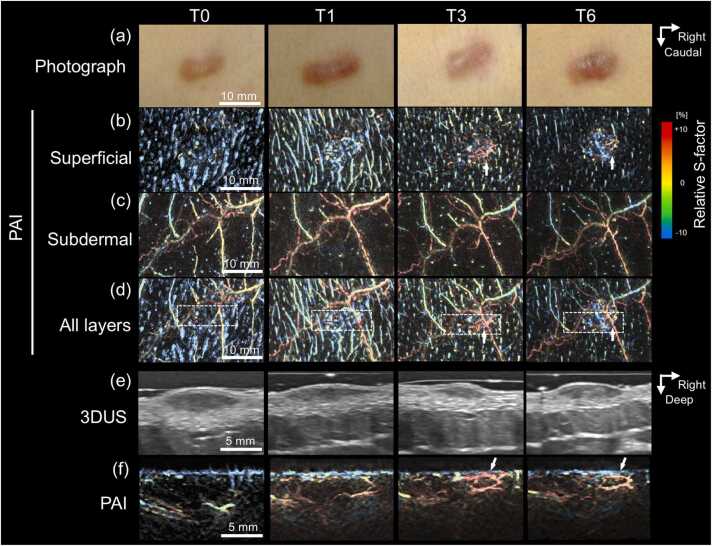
Longitudinal changes in Case 3. The keloid initially exhibited a small, oval morphology (a) with a lenticular profile on ultrasonography (e). The lesion flattened between T0 and T1; however, thickening was observed between T3 and T6. Photoacoustic images with color-coded relative S-factor values reveal no clusters of microvessels in the superficial layer (b), whereas several veins with heterogeneously elevated S-factor levels are present in the subdermal layer (c). After recurrence, thickened superficial microvessels with elevated oxygen levels are observed (b, d, arrows). Sectional images corresponding to rectangular regions in (d) demonstrate curved continuity between these superficial vessels and the subdermal vascular network along the keloid periphery (f, arrows).
